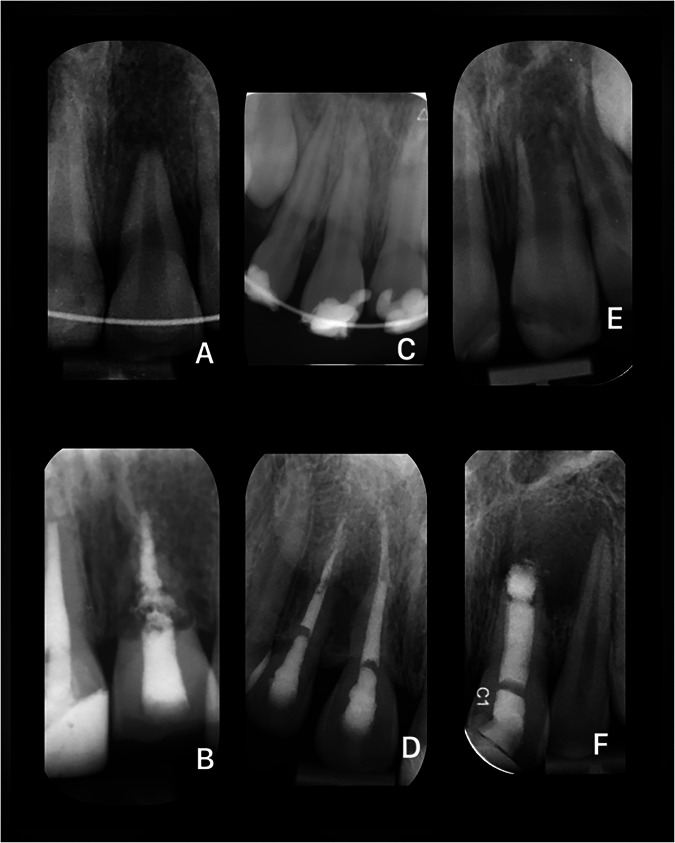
Pre- and post-operative radiographs of example cases with an “Unfavourable” outcome. A Maxillary central incisor in a patient between 10 and 15 years old with a history of avulsion (Pre-op). B The same tooth at 53 months post-treatment. C Maxillary central and lateral incisors in a patient between 10 and 15 years old with a history of avulsion (Pre-op). D The same teeth at 5 months post-treatment. E Maxillary central incisor in a patient between 5 and 10 years old with a history of enamel-dentine fracture (Pre-op). F The same tooth at 43 months post-treatment.
